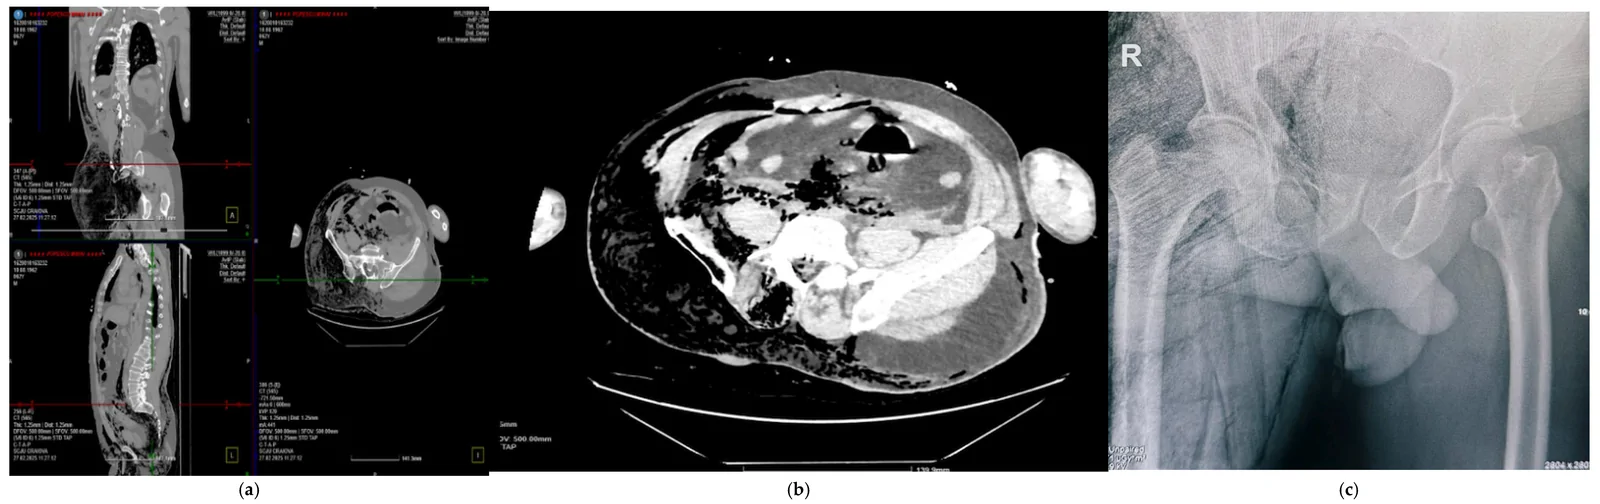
Imaging findings in a patient with fasciomyonecrosis: (a,b) computed tomography (CT) images demonstrating the extent of the disease; (c) plain radiograph (X-ray).
